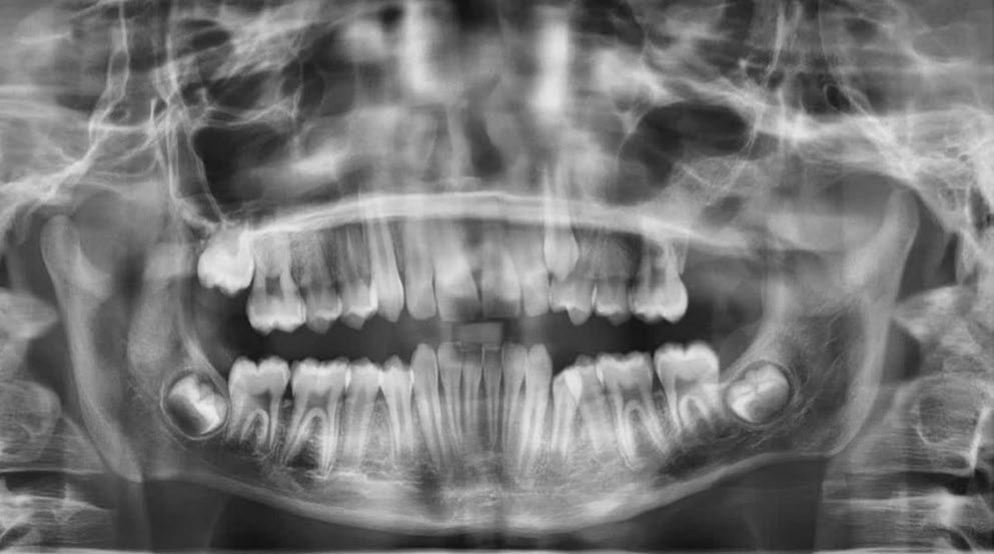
Panoramic radiograph taken at 5‐month follow‐up, demonstrating complete healing of the surgical site with no evidence of recurrence.
